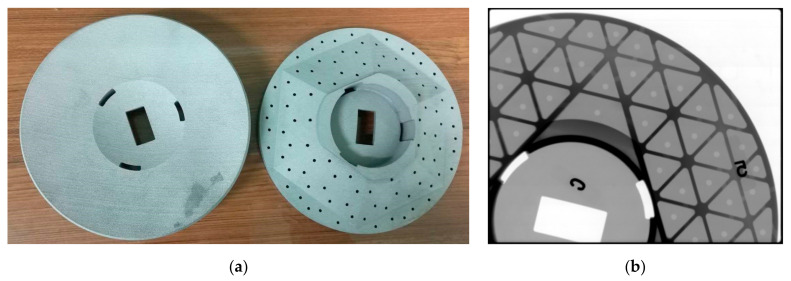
(a) AM mirror blanks after cleaning; (b) X-ray inspection image for the AM mirror blank (the letter and number are locating datum).
